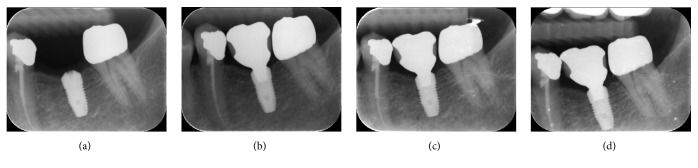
Radiographic variations during 12 months after loading in platform-switched dental implants. (a) Baseline, before loading; (b) immediately after loading; (c) 6 months after loading; (d) 12 months after loading.
